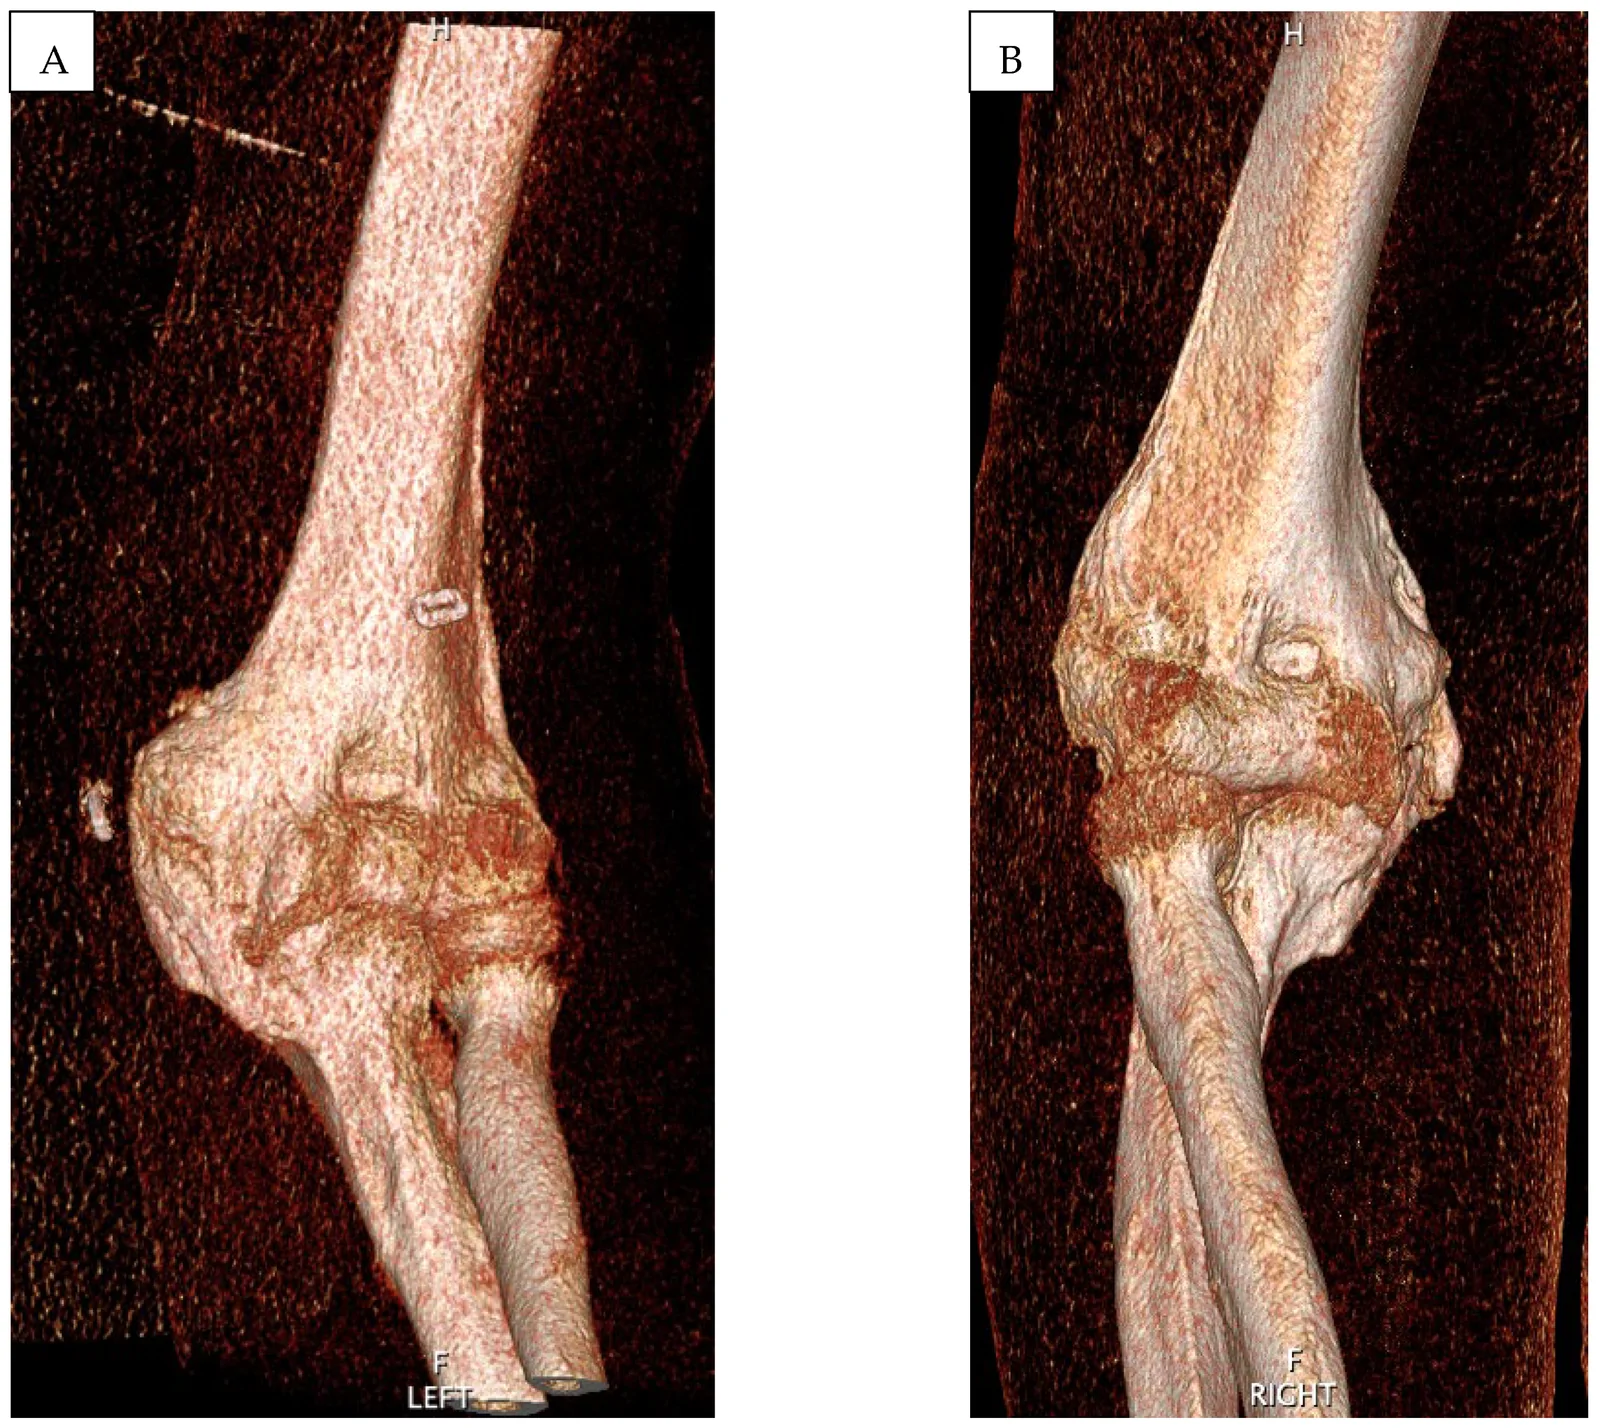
Preoperative (A,B) 3D computed tomography renderings demonstrating severe heterotopic ossification about the left and right ulnohumeral joints, respectively. These images were used preoperatively to guide treatment.
